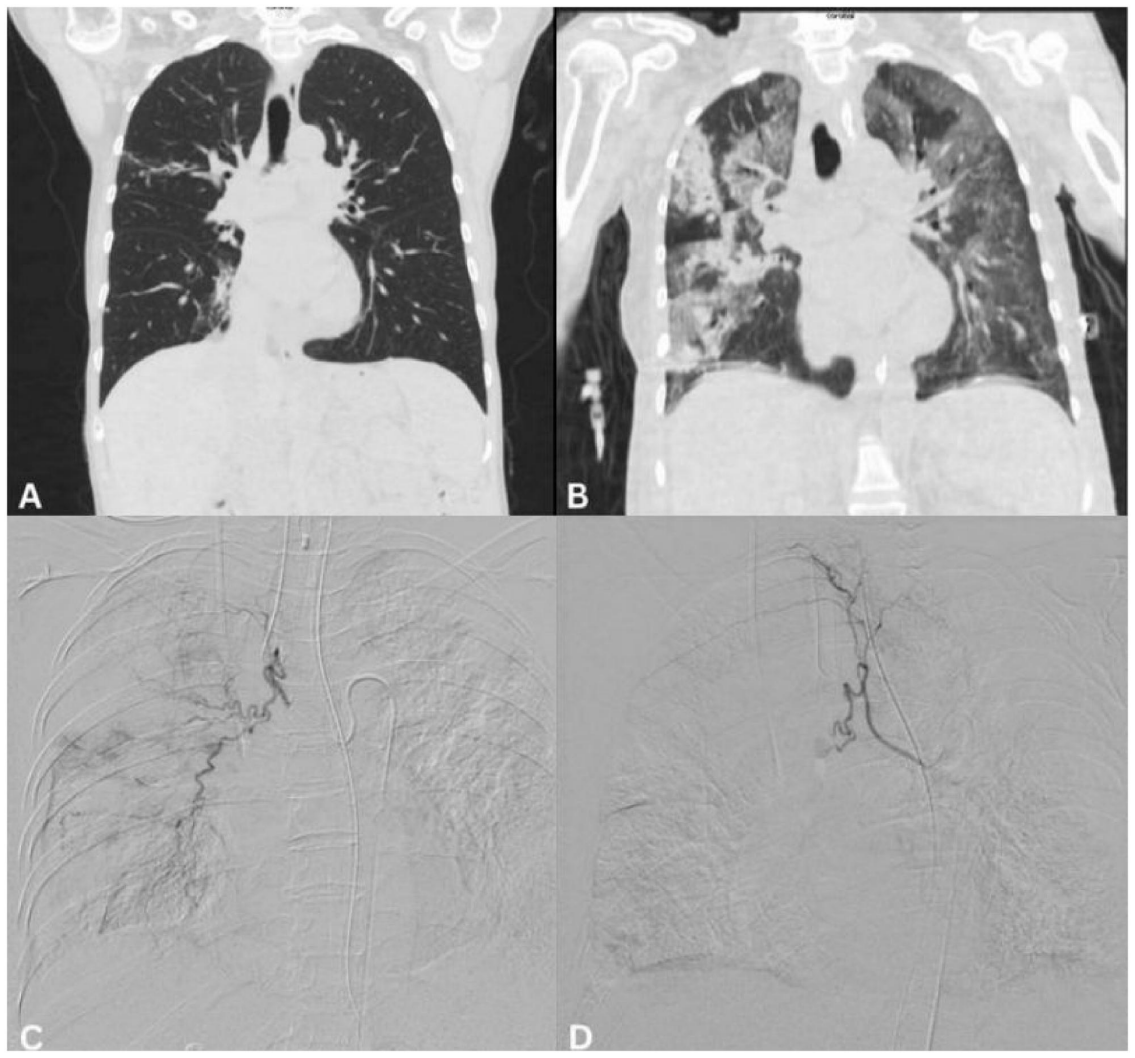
Embolization of haemoptysis in a leukaemia patient. 65-year-old female with chronic lymphocytic leukaemia. (A) Baseline coronal CT image demonstrates minor areas of atelectasis in the right upper and middle lobes (20 months before the date of embolization). (B) Coronal CT image, acquired during the admission for haemoptysis, demonstrates bilateral patchy ground-glass and consolidative opacities with a greater preponderance in the right lung. (C) Following bronchoscopy (which suggested evidence of bleeding from the right lung), selective angiography of the right bronchial artery demonstrated arterial hypertrophy and prominent parenchymal vascularity, correlating with CT and bronchoscopic findings. (D) The artery was then embolized to near-stasis with 100-300 and 300-500 micron Embospheres (Merit Medical, South Jordan, UT, USA), with significant reduction in parenchymal vascularity. Despite technical success and cessation of haemoptysis, the patient died 13 days later from progressive respiratory failure.
